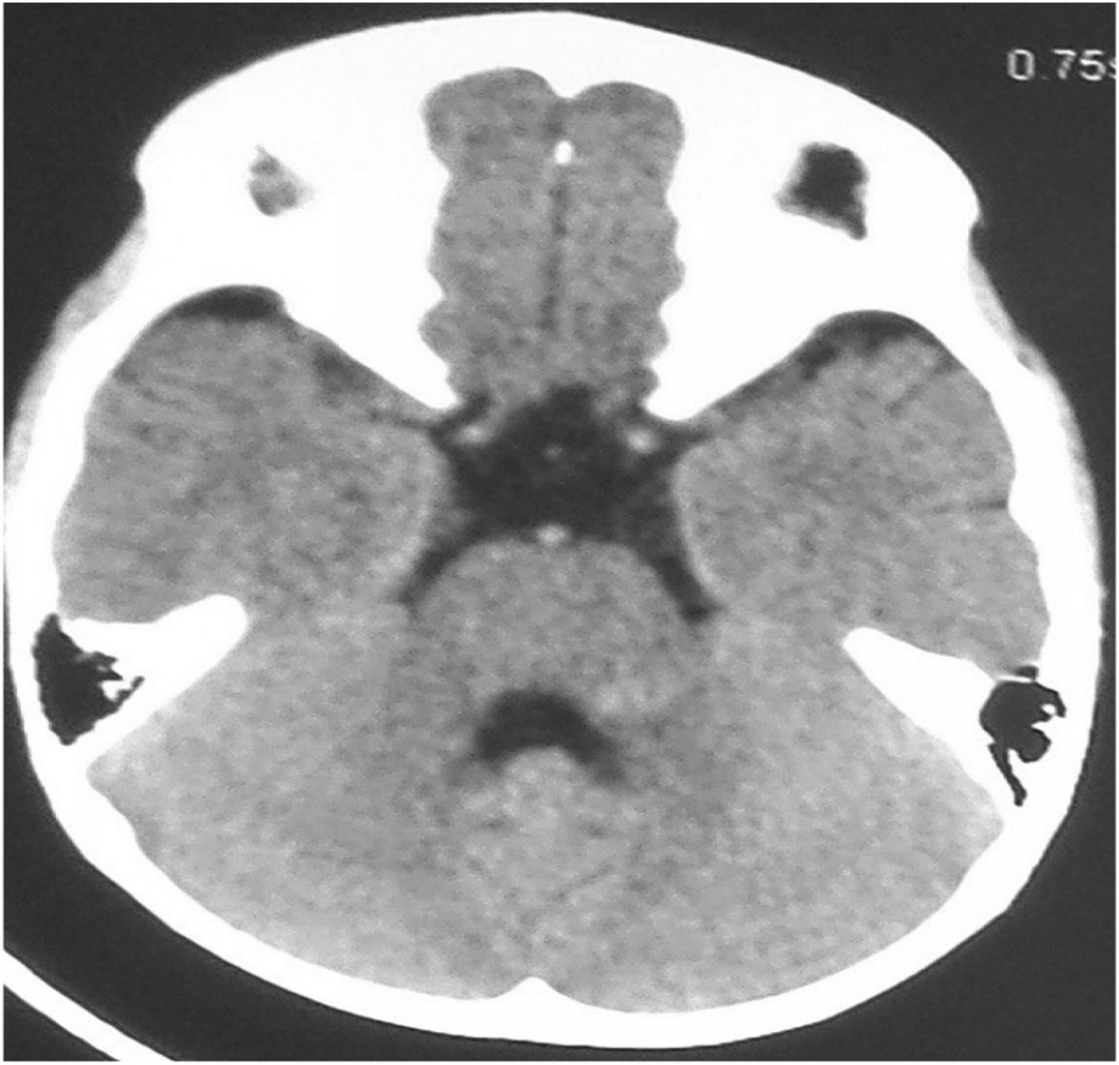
Non‐contrast CT brain, axial slice performed 7 days after the initial CT showing complete resolution of the abnormality.
